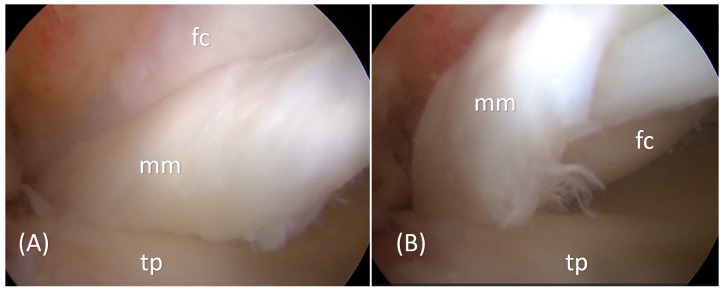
Arthroscopic evaluation of the left knee confirmed a displaced bucket-handle tear of the medial meniscus. Intraoperative views (Panels (A,B); mm: medial meniscus, tp: tibial plateau, fc: femoral condyle) demonstrate the displaced meniscal fragment folded into the intercondylar notch, causing mechanical obstruction and corresponding to the double PCL sign observed on preoperative MRI. The arthroscopic findings directly correlate with the preoperative MRI appearance, with the displaced meniscal fragment visualized intraoperatively in the same anatomical relationship to the intact PCL as identified on imaging, thereby confirming the radiologic diagnosis. The torn fragment was unstable, located predominantly within the red–white zone, and demonstrated chronic displacement, rendering meniscal repair less favorable due to limited healing potential. In addition, the fragment showed deformation and poor tissue quality, and was not adequately reducible to its native position, further limiting the feasibility of repair. Therefore, arthroscopic partial meniscectomy was performed to remove the displaced fragment and restore joint mechanics. Postoperatively, the patient followed a standard rehabilitation protocol without case-specific modifications and returned to full athletic activity within 12 weeks. At one-year follow-up, the patient reported complete resolution of symptoms, had returned to full athletic activity without limitations, and clinical examination demonstrated a painless knee with full range of motion (0–140°), absence of joint-line tenderness, and negative McMurray and Thessaly tests, indicating an excellent functional outcome. Meniscal preservation is generally preferred whenever feasible, given its role in load distribution, joint stability, and cartilage protection [10]. Current trends increasingly favor meniscal repair, even in complex tear patterns, supported by evidence demonstrating lower rates of progression to advanced osteoarthritis and subsequent knee arthroplasty compared to partial meniscectomy [11]. However, healing potential is closely related to vascular supply, which is largely confined to the peripheral red–red zone, while the inner regions demonstrate reduced vascularity [12]. In the present case, the tear was located predominantly within the red–white zone and showed chronic displacement and deformation, likely related to delayed presentation, reducing the likelihood of successful repair [13]. Therefore, partial meniscectomy was considered the most appropriate option to relieve mechanical symptoms and restore knee function. Of course, given the single-case nature of this report, the clinical outcome should be interpreted in the appropriate context. Although this approach is associated with recognized long-term risks, careful and limited resection remains an accepted treatment strategy when repair is not feasible [13,14,15].
